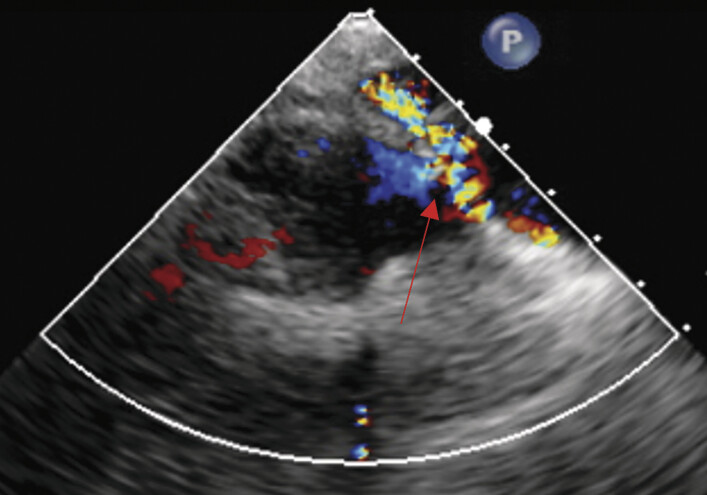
Intraoperative transesophageal echocardiography showing the aortopulmonary shunt.
